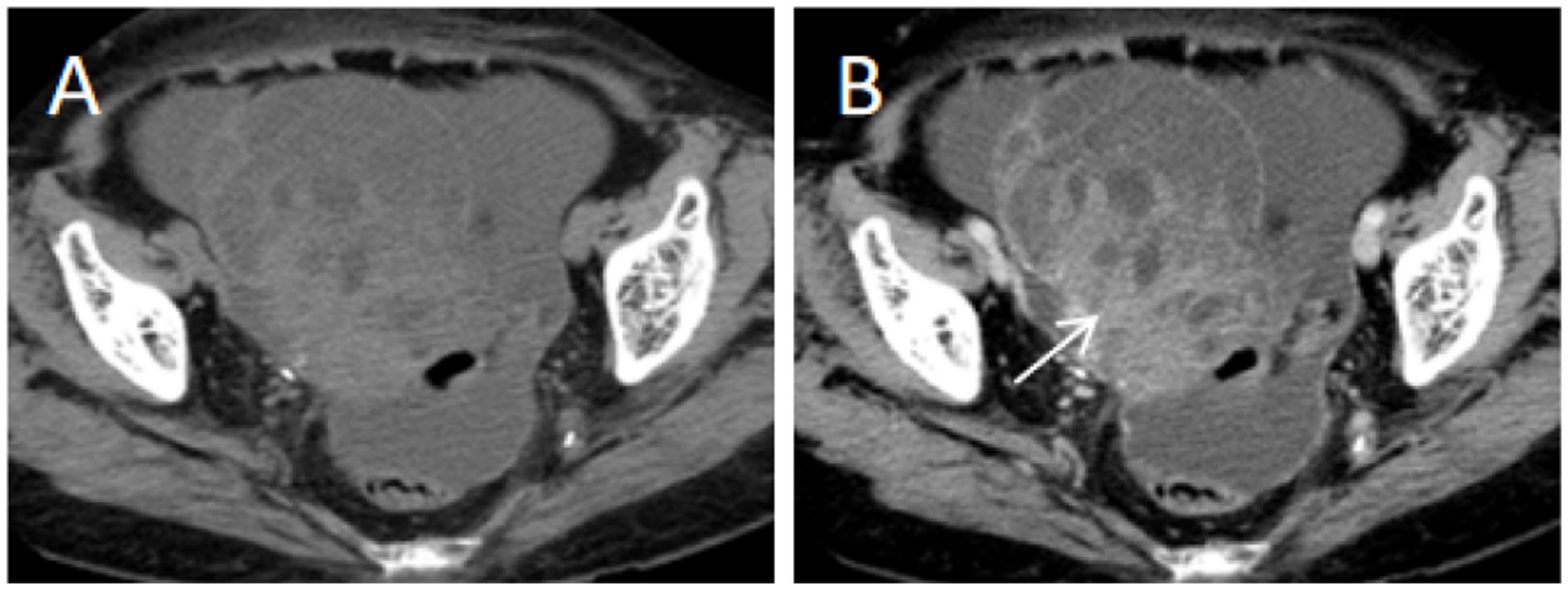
91-year-old patient with right ovarian mucinous adenocarcinoma (malignant). (A) Axial unenhanced CT image shows a cystic-solid mass in the right ovary, with multiple thickened septa (thickness ≥4 mm). (B) Axial contrast-enhanced CT image (portal venous phase) demonstrates prominent enhancement of the solid components (arrow). Scoring rationale: CA125 level was elevated (289.5 U/mL, 3 points), texture was cystic-solid (5 points), septum thickness ≥4 mm (1 point), enhancement degree was prominent (1 point). Therefore, a score of 10 was assigned for this patient.
